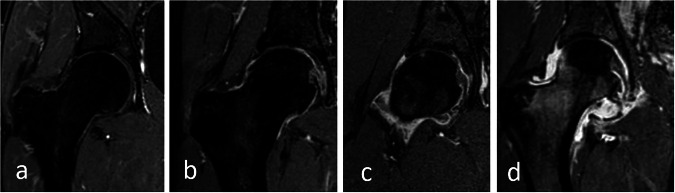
Hip MRI (T1-weighted, fat-saturated post-contrast images) in four different children aged 12 years, showing different grades of synovial enhancement: (a) normal, (b) mildly increased synovial enhancement, (c) moderately increased, and (d) severely increased synovial enhancement
